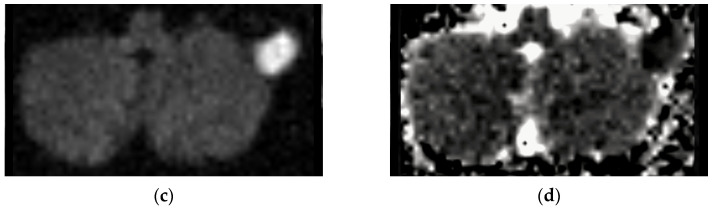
Thirty-seven-year-old man with chronic otitis media and history of ear surgery. MRI T1w (a) and contrast-enhanced T1w (b) sequences showing hypo-isointense material in the left mastoid (black arrows) spreading in the neck, in the posterior cervical space (white arrowheads). Note the mastoid mucosal enhancement, suggestive for mastoiditis, and the peripheral enhancement of the neck component (b). The material in the mastoid is hyperintense in diffusion-weighted imaging (c) and shows inhomogeneous apparent diffusion coefficient values (d), consistent with purulent collection and cholesteatoma. Bezold’s abscess and left mastoid cholesteatoma were confirmed at surgery.
